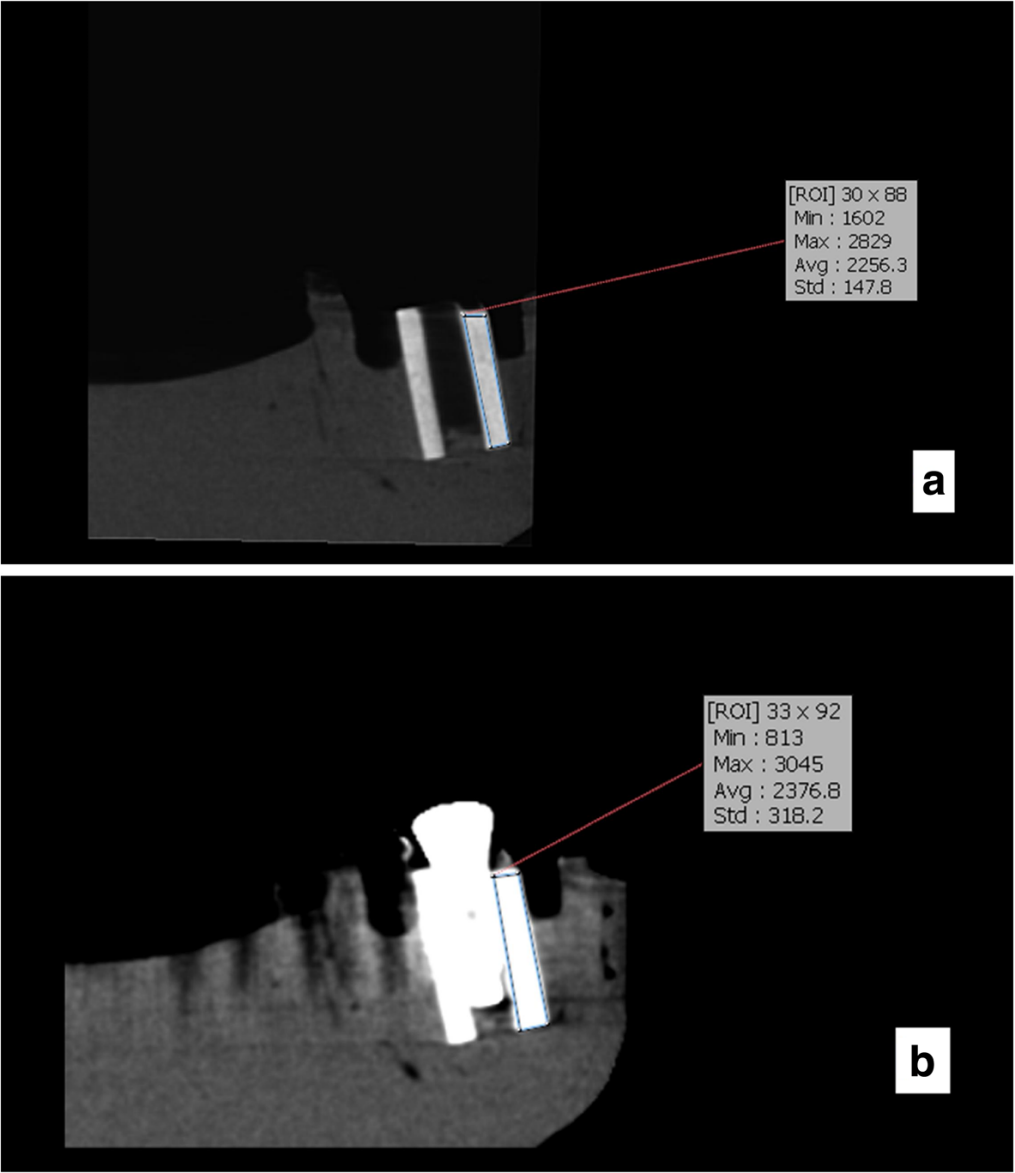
CBCT scans of the samples for type 4 bony density before (a) and after (b) insertion of implants in this exposure parameters: FOV: 6 × 8 cm^2^, mA: 10, T:12.6 s,kVp:90
